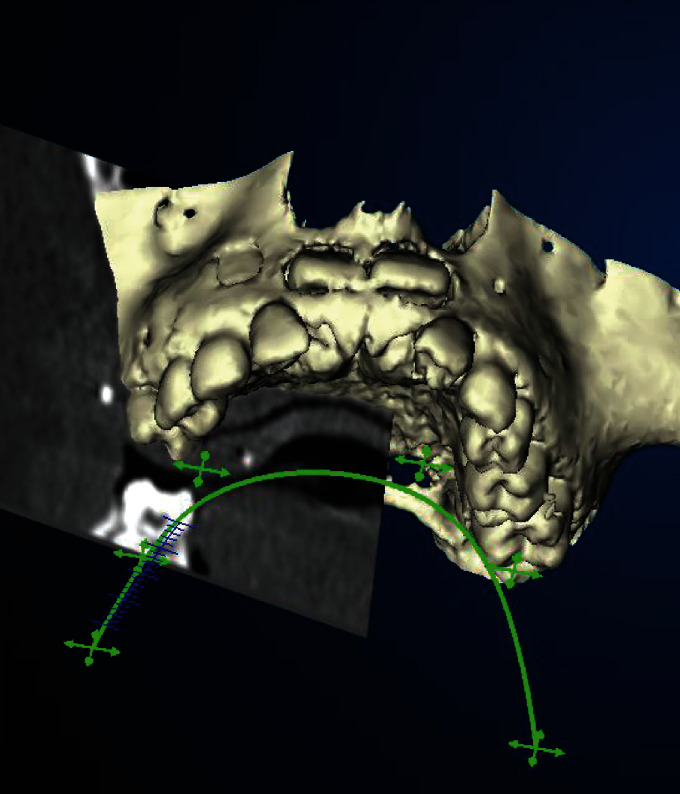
Digital volume tomography; CB-CT (3D-Kavo Exam) in the caudolaterocranial view with projected upper jaw line (green) and transversal section plane.
